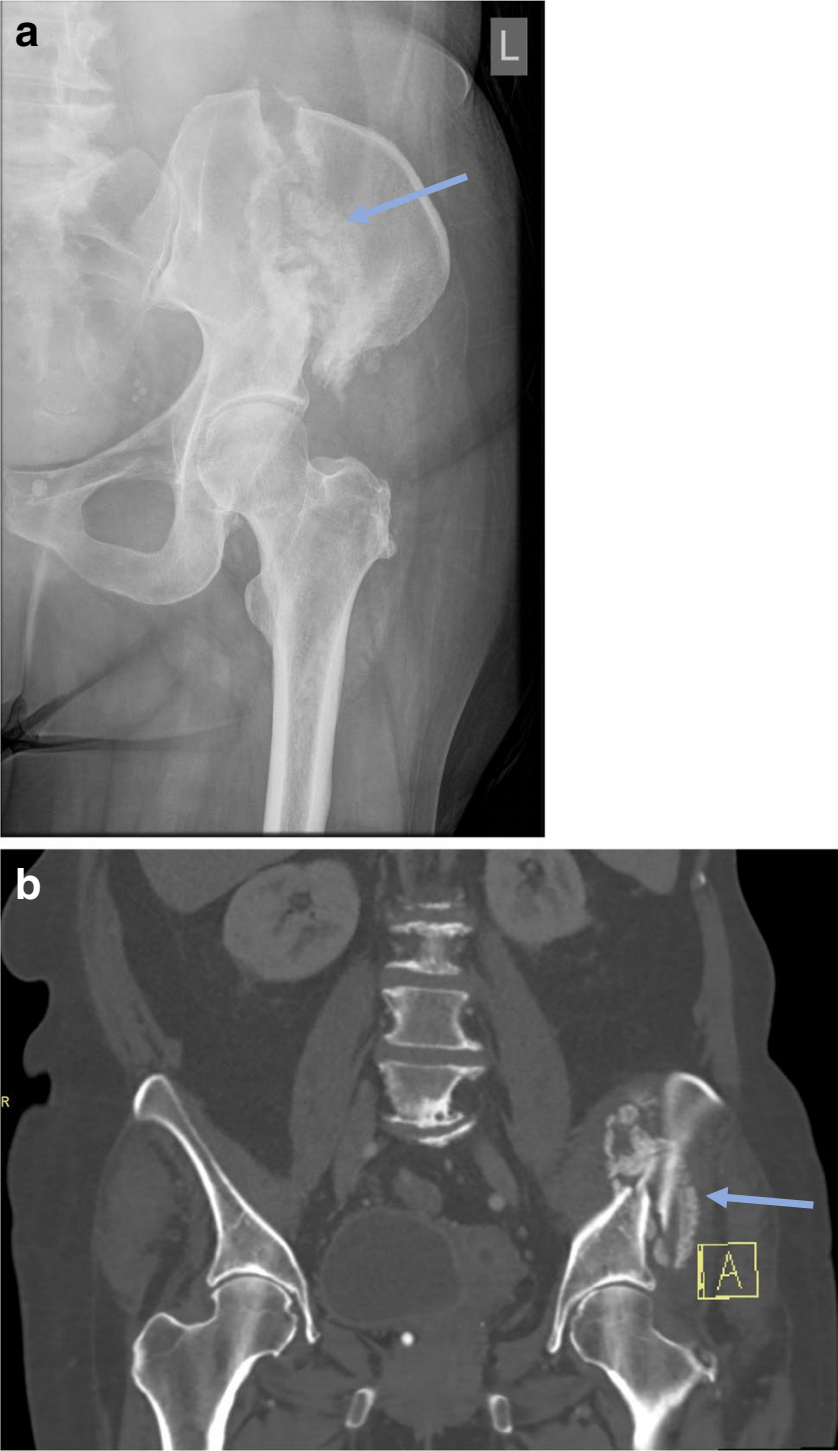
A left iliac wing fracture (blue arrow) associated with bisphosphonate use in a 63-year-old after 2 months. Anteroposterior pelvic radiograph showing florid callous formation at the fracture site (a) and corresponding coronal computed tomography of the abdomen and pelvis showing external callous formation (b)
